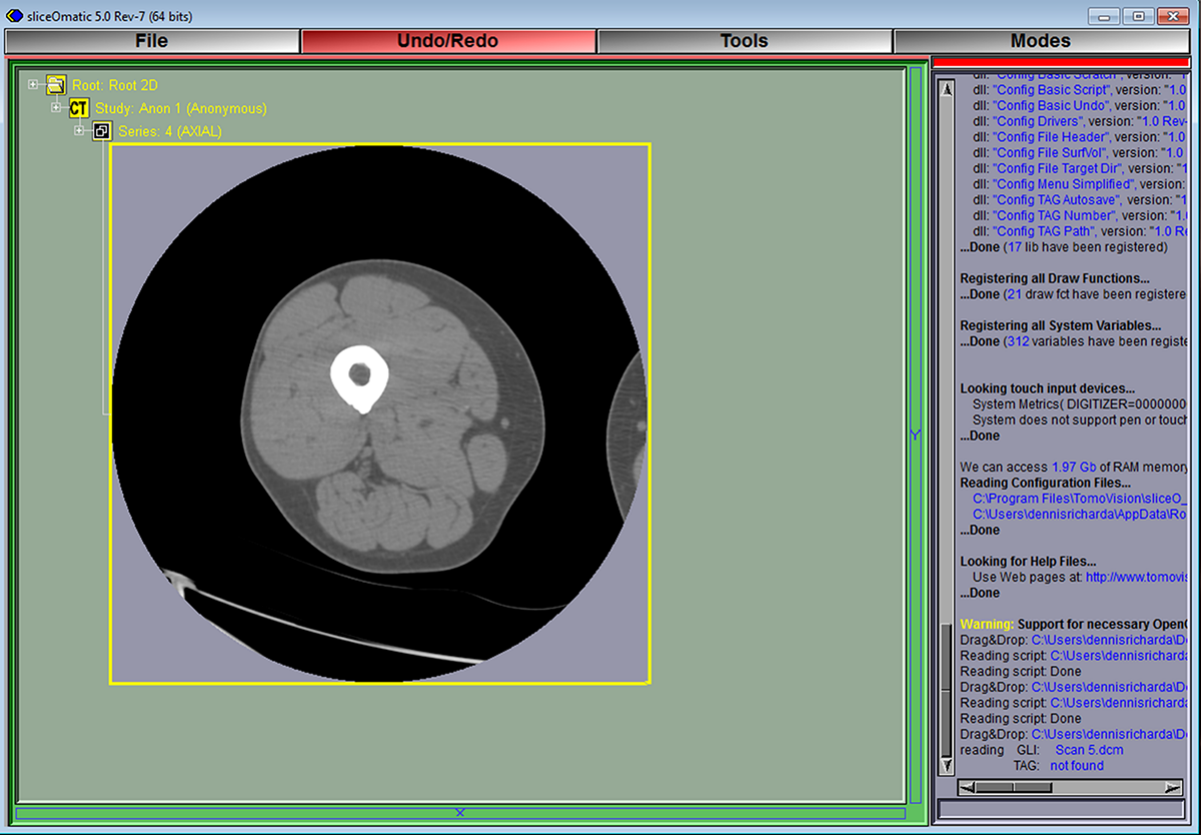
The CT grey level images or DICOM files should be available in a convenient known location such as a desktop folder. A CT scan is opened by dragging the image file into the Display Area. SliceOmatic can open multiple images at the same time but it is recommended that the user focuses on single images until familiar with the program and the analysis method.
